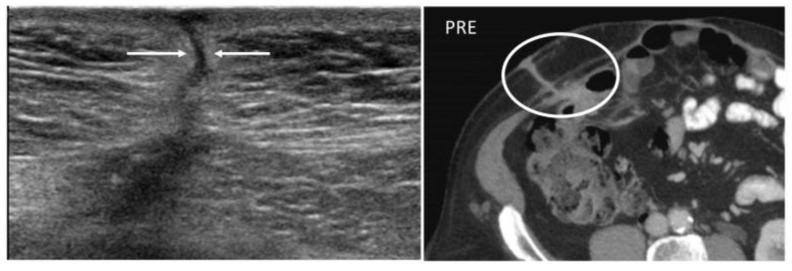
A 22-year-old man with reactivation of Crohn’s disease, presenting as a new enterocutaneous fistula. B-mode US with linear transducer (first picture) shows the presence of a fistulous trait between the skin and a superficial collection (arrows). Axial not-enhanced CT scan (second picture) revealed the course of the fistula, demonstrating communication between the bowel loops and the skin, together with a small collection (circle).
